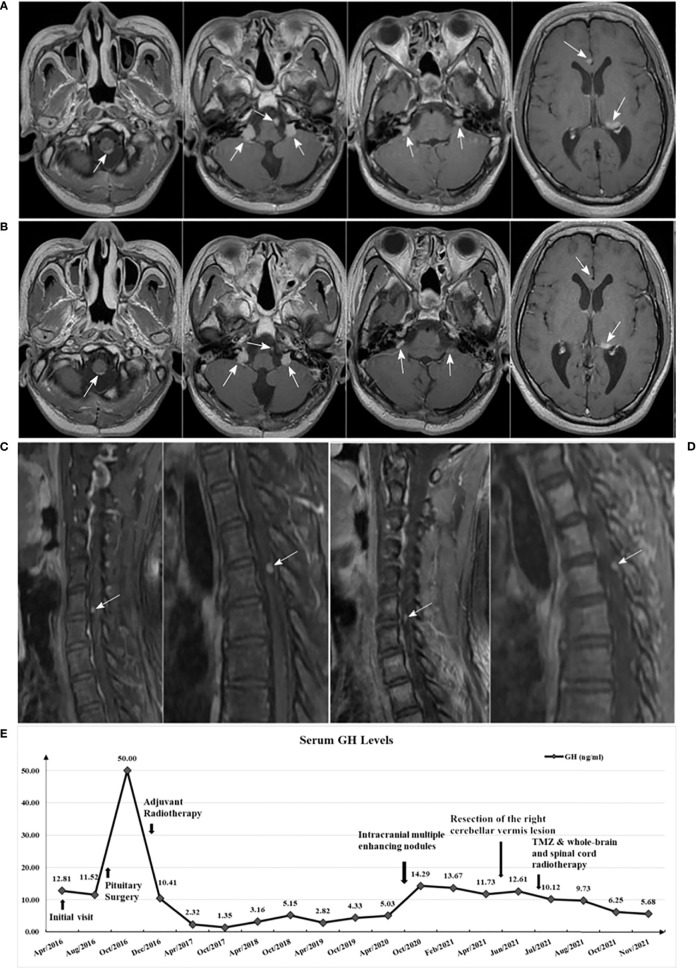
(A) Brain enhanced MRI in May 2021 (before treatment); (B) Brain enhanced MRI in November 2021 (after treatment) showed: multiple lesions all reduced in size compared with A; (C) Spinal cord enhanced MRI in June 2021 (before treatment); (D) Spinal cord enhanced MRI in November 2021 (after treatment) showed: enhanced nodules in the sixth cervical vertebrae and fourth thoracic vertebral plane of the spinal cord both mildly decreased compared with C; (E) Serum GH levels variation curve of the patient.
